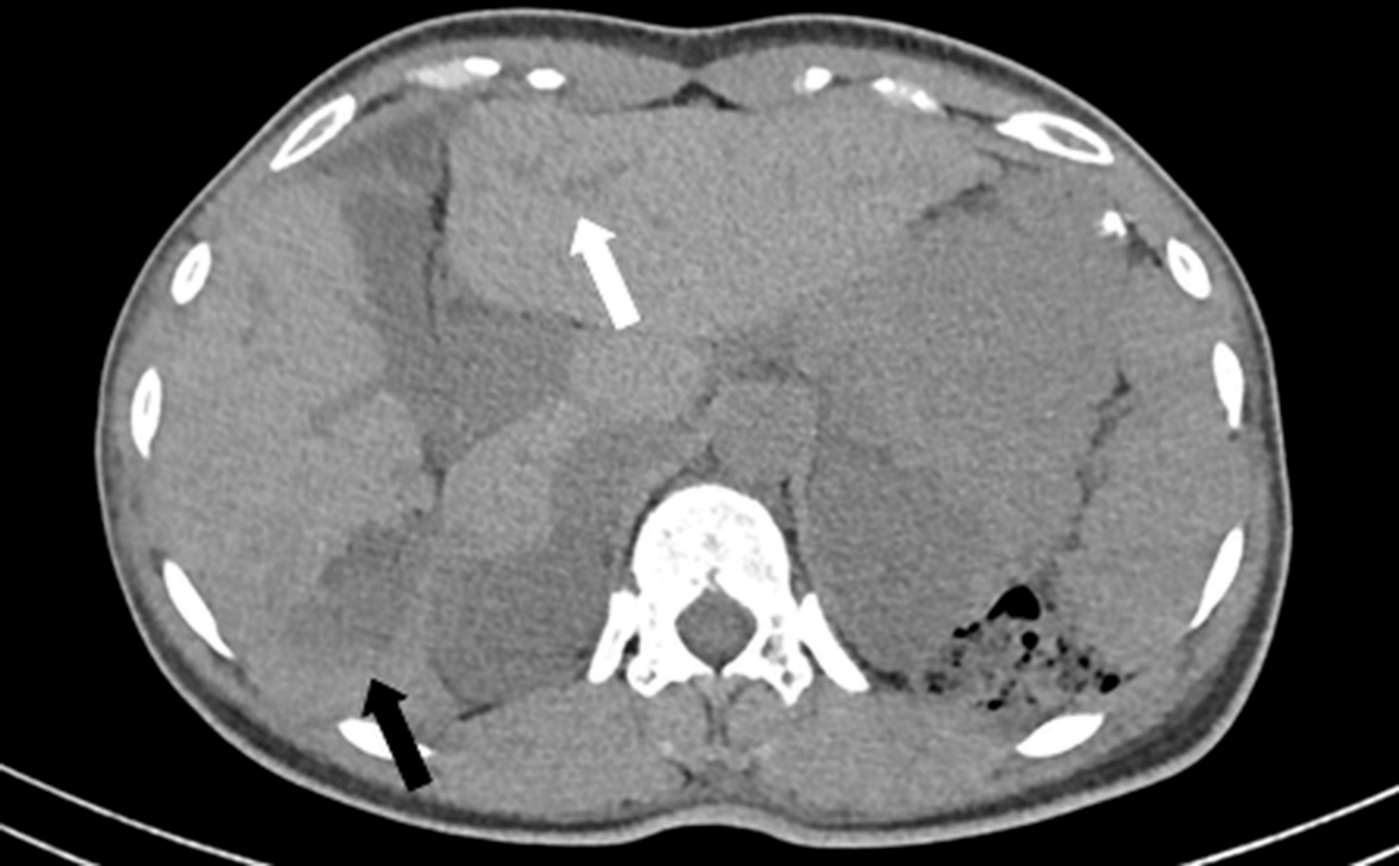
Axial non-enhanced CT image at the level of the liver Axial non-enhanced CT image at the level of the liver demonstrating a globular lesion in segment VI that appears relatively hypodense compared with the surrounding liver parenchyma and measures 4.5 cm x 2.7 cm. The lesion shows heterogeneous attenuation with areas of relatively higher density (black arrow). Irregular dilatation of the intrahepatic bile ducts is also visible (white arrow).
